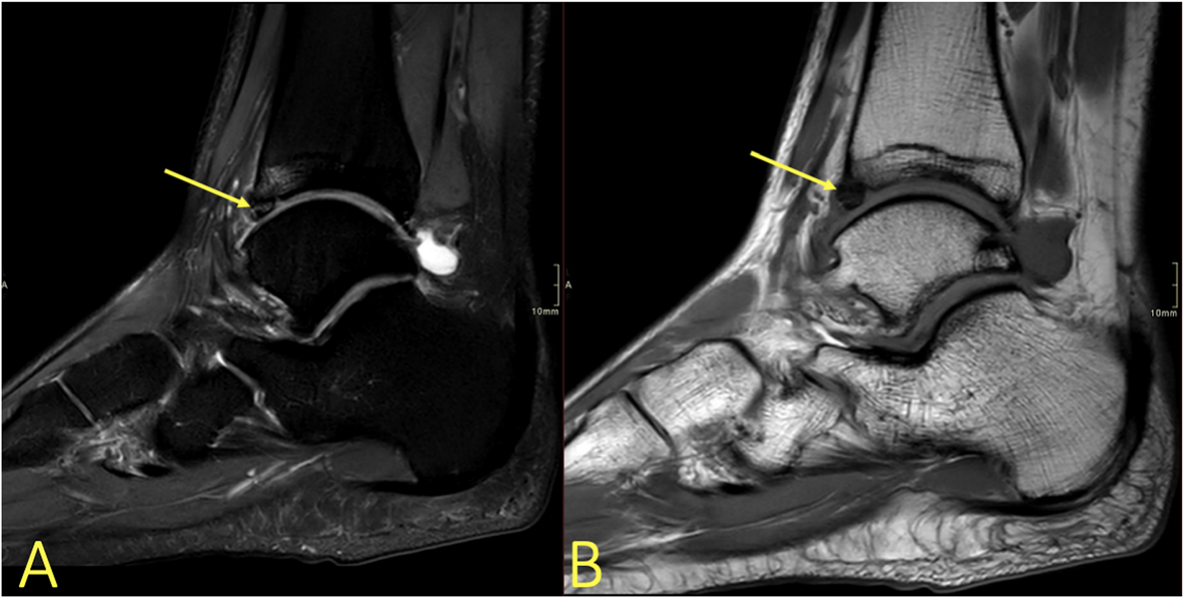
A 55-year-old male with chronic anterior ankle pain. He had had a previous ankle injury about 8 years ago without fracture but now suspicion of developing osteoarthritis. MRI showed osteophytes at the anterior border of the distal tibia with bone marrow edema (arrows) showing anterior impingement
